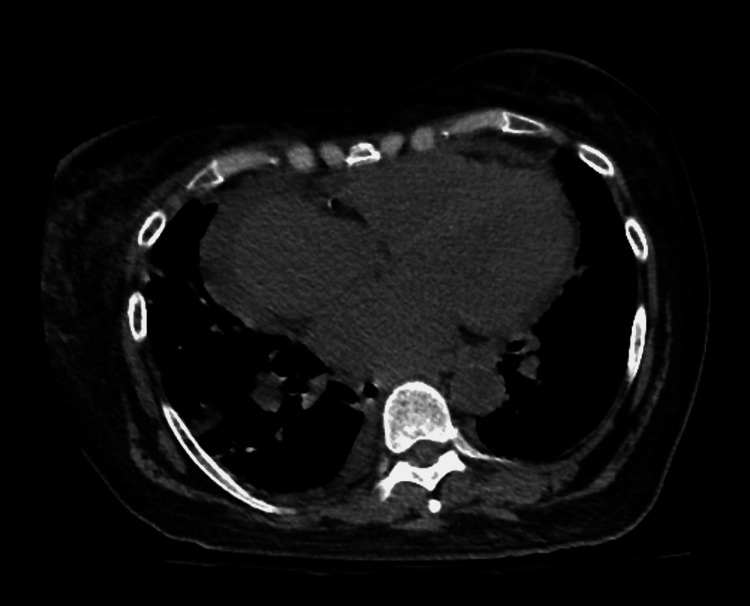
Chest CT 3 weeks before patient presentation. Cardiomegaly with no pericardial effusion CT, computed tomography.
